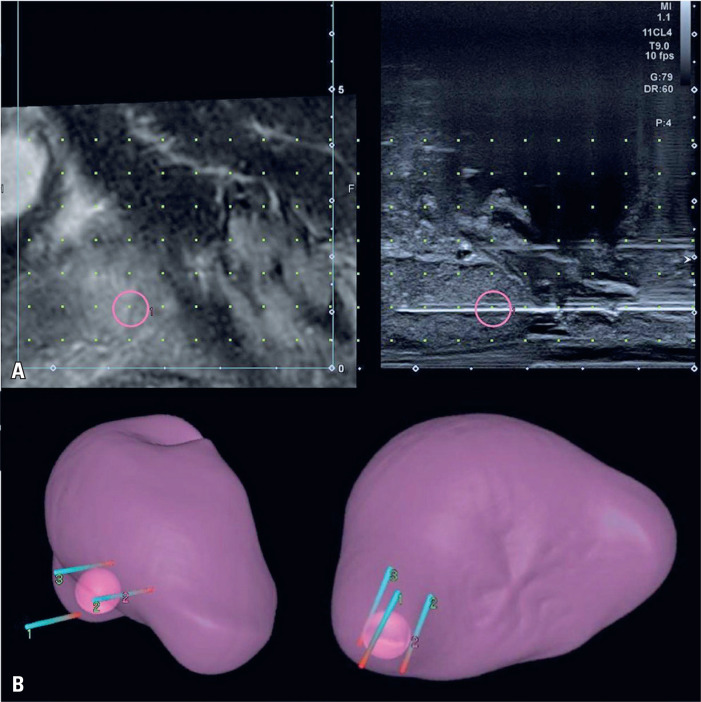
Transperineal biopsy. A) A real-time fusion of the prostate MRI coupled with the sagittal view of the transrectal ultrasound during the transperineal biopsy of the nodule. B) A 3D reconstruction depicts the position of the electroporation probes relative to the prostatic nodule
